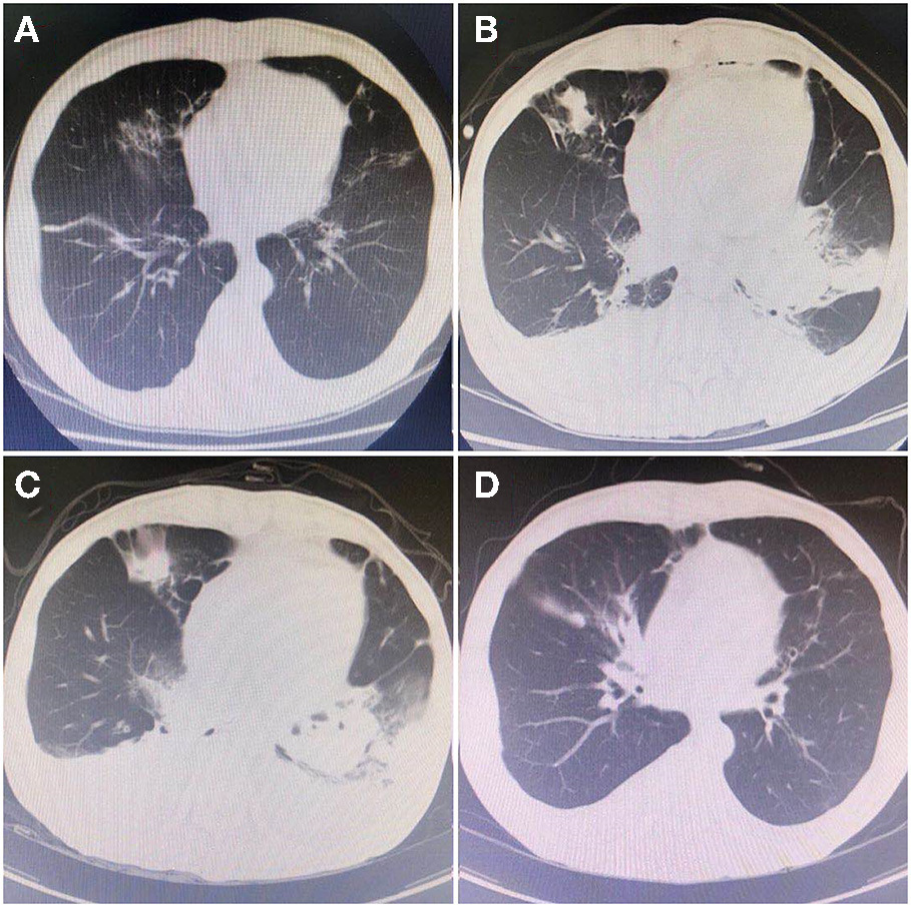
Chest CT indicated dynamic changes during the infections. (A) On May 13, 2020, preoperative examination of pulmonary: multiple chronic or old lung lesions. (B) On May 31, 2020, the first CT during infection: showed patchy high-density shadows in the basal segment of the lower lobes of both lungs. (C) On June 2, 2020, the second CT: range of high-density shadows was more obvious than the previous. (D) On June 10, 2020, the last CT: high-density shadows gradually disappeared, which were greatly improving compared with the previous.
